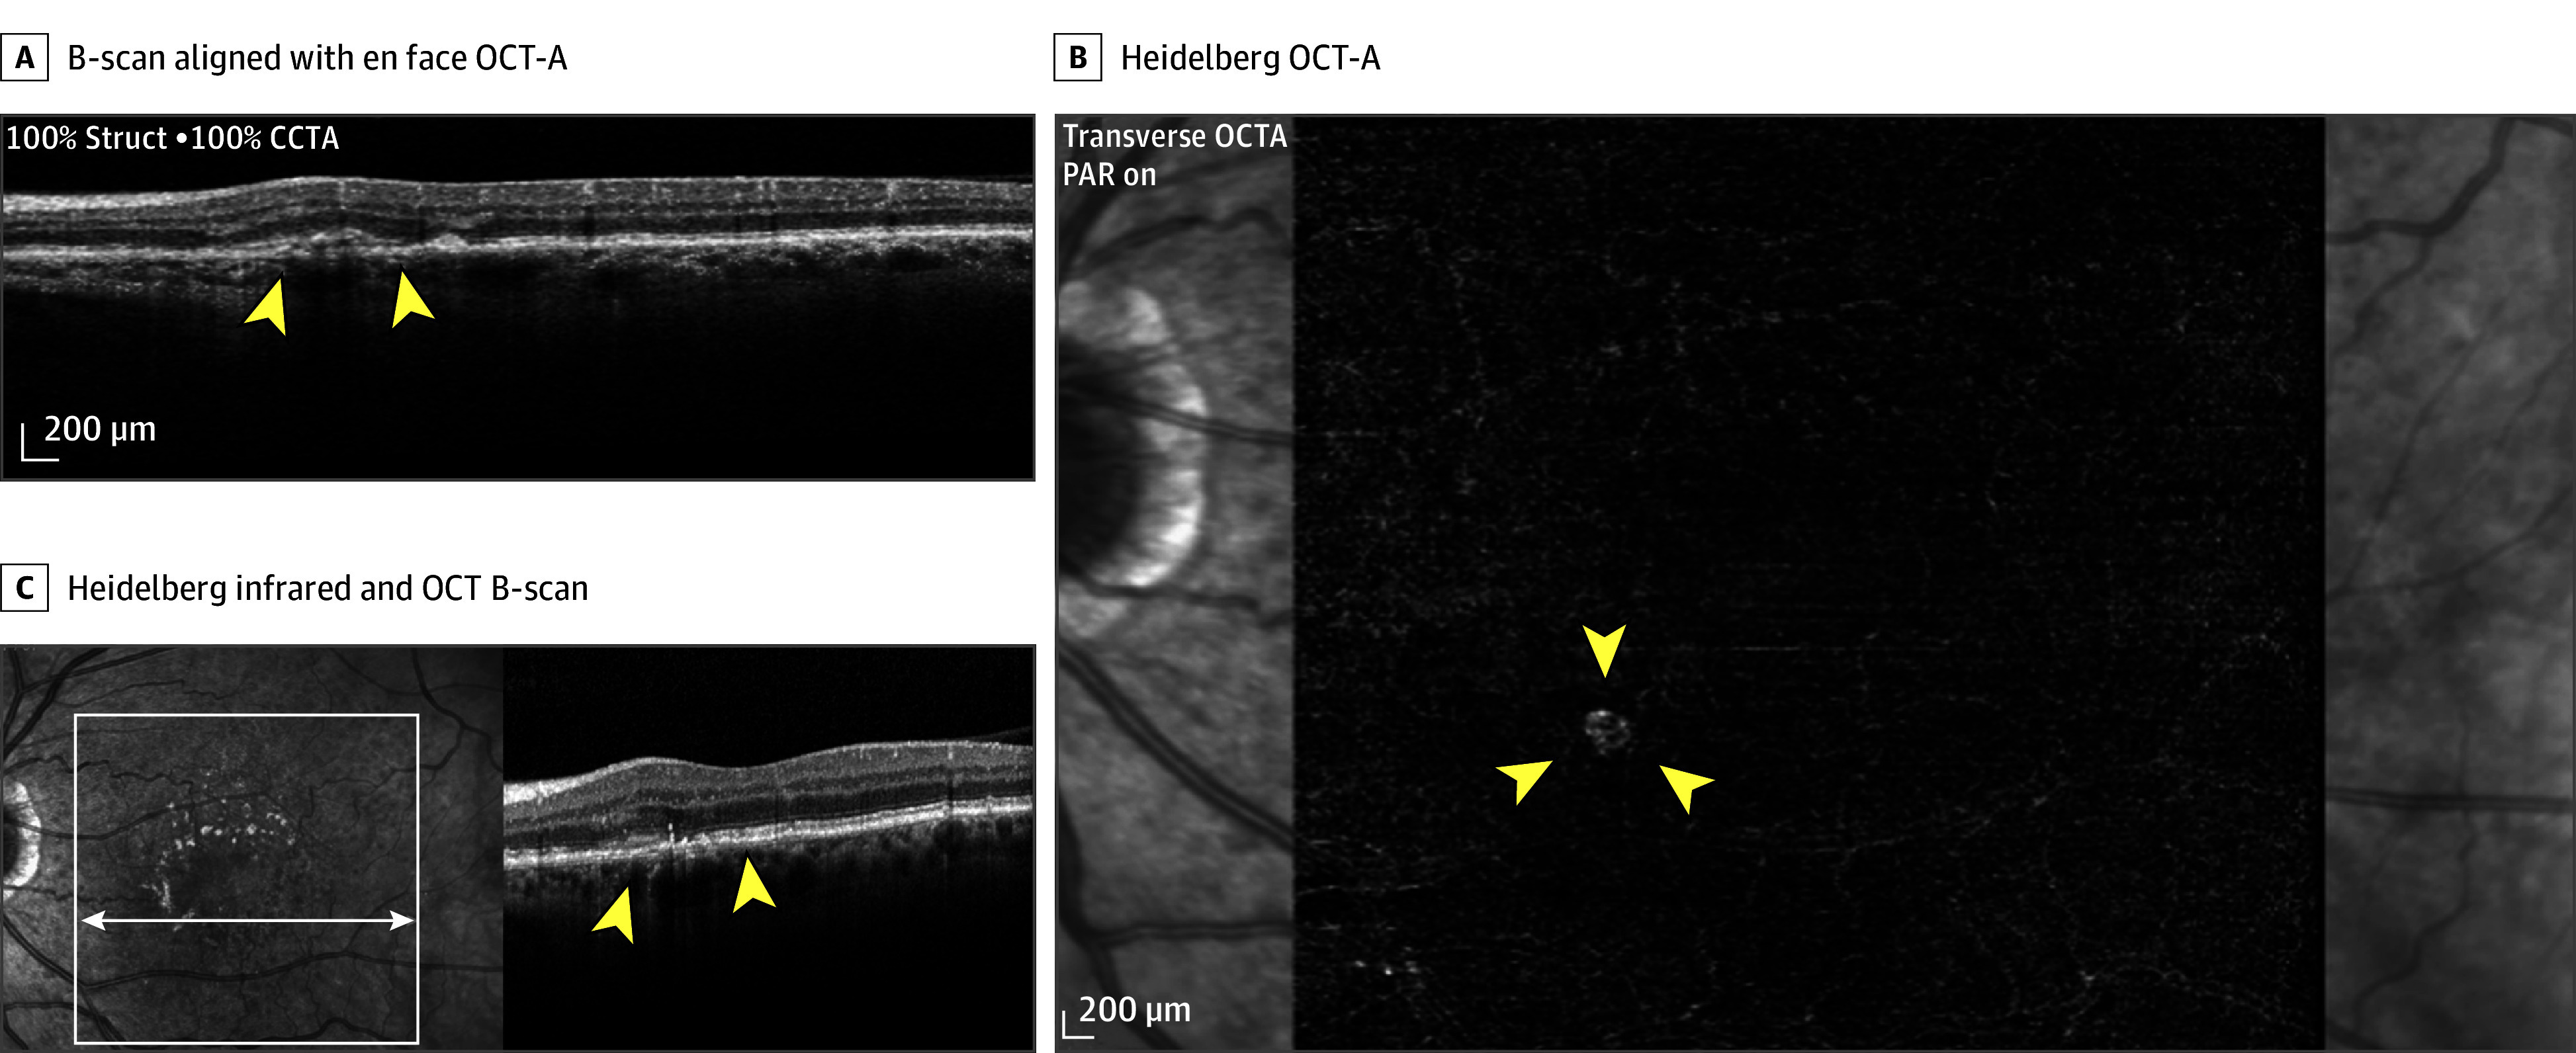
Imaging Showing a Thin Double-Layer Sign That Contains a Nonexudative Neovascular Membrane A, The brightness scan (B-scan) that aligns with the enface optical coherence tomography angiography (OCT-A) image and the boundary is indicated by yellow arrowheads. B, Heidelberg OCT-A showing the nonexudative neovascular membrane and the boundary of choroidal neovascularization is indicated by yellow arrowheads. C, Heidelberg infrared and OCT B-scan showing the double-layer sign and the boundary is indicated by yellow arrowheads. CCTA indicates coronary computed tomography angiography; PAR, persistent avascular retina.
